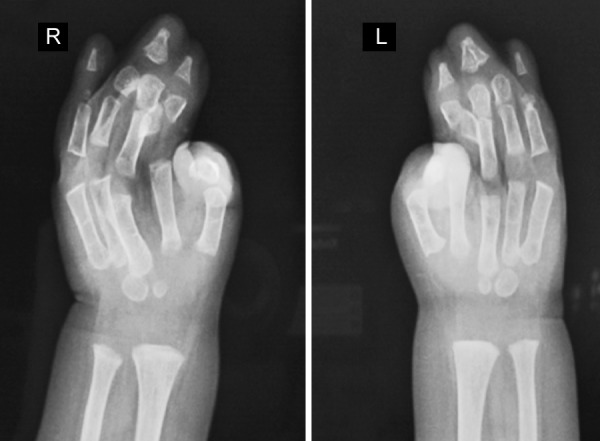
Hand-wrist radiograph showing soft tissue syndactyly of all the digits and synostosis involving phalanges of second, third and fourth digits and metacarpels of both the hands
